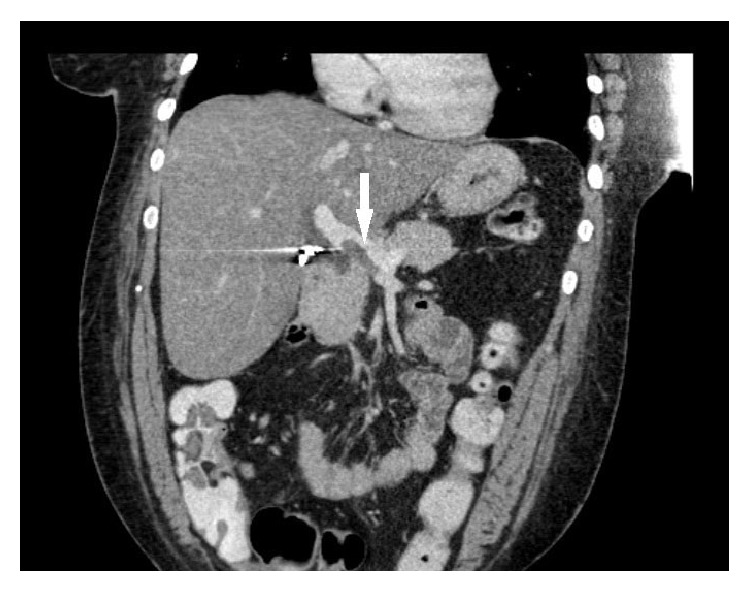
Computed tomography of abdomen and pelvis coronal view showing portal vein thrombosis.
